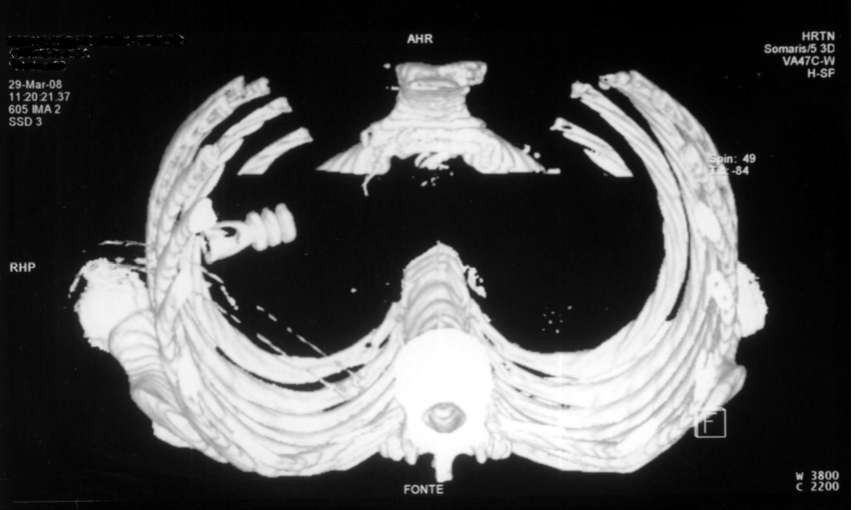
Admission CT scan of the chest. CT three-dimensional (3D) image reconstruction of the chest shows an intra-thoracic attenuated energy projectile and a chest thoracostomy tube inside the right hemithorax.
